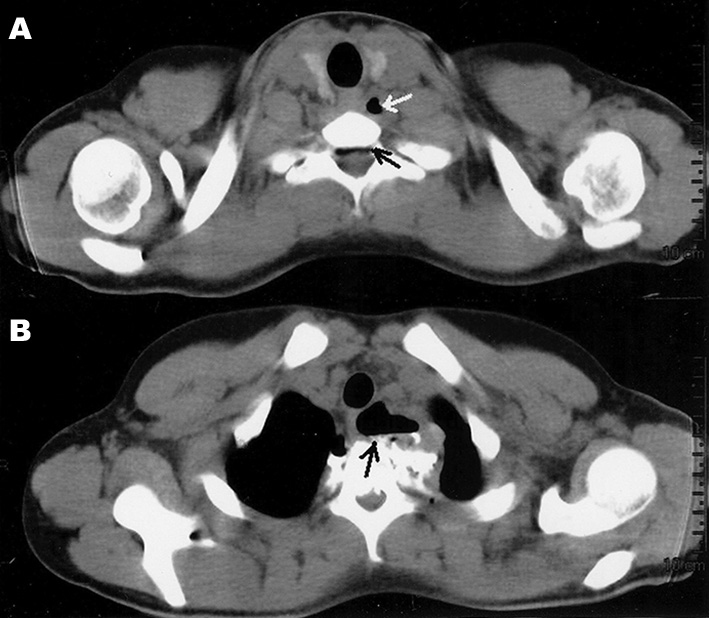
A: CT scan showing neck extraluminal air (clear arrow) and air in the spinal canal (dark arrow). B: Extensive air collection
